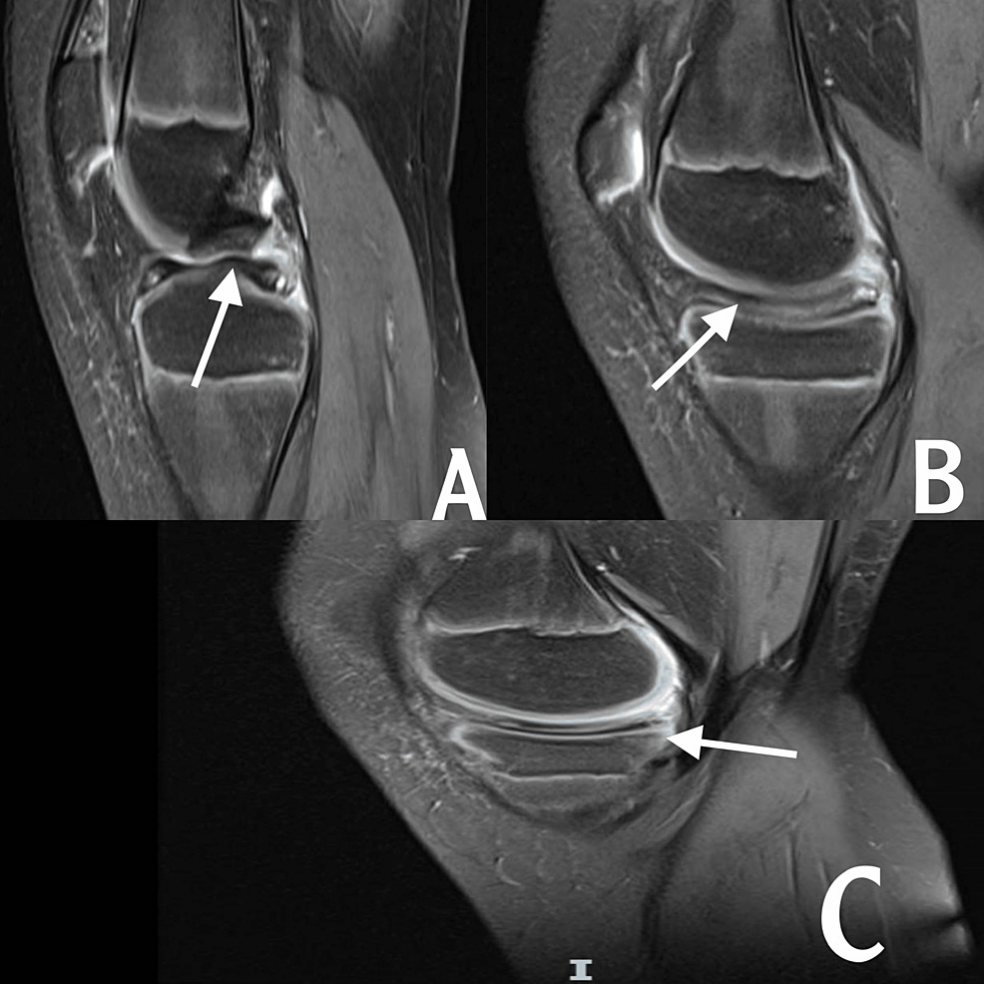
Left knee MRI sagittal view
